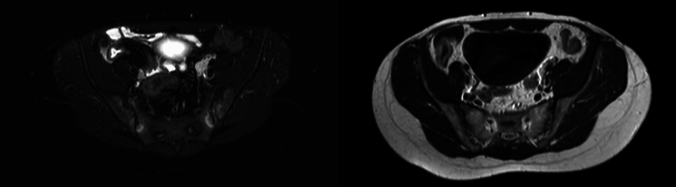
Axial MRI scans of the sacroiliac joints show bilateral sacroiliitis, with subchondral bone marrow edema appearing
